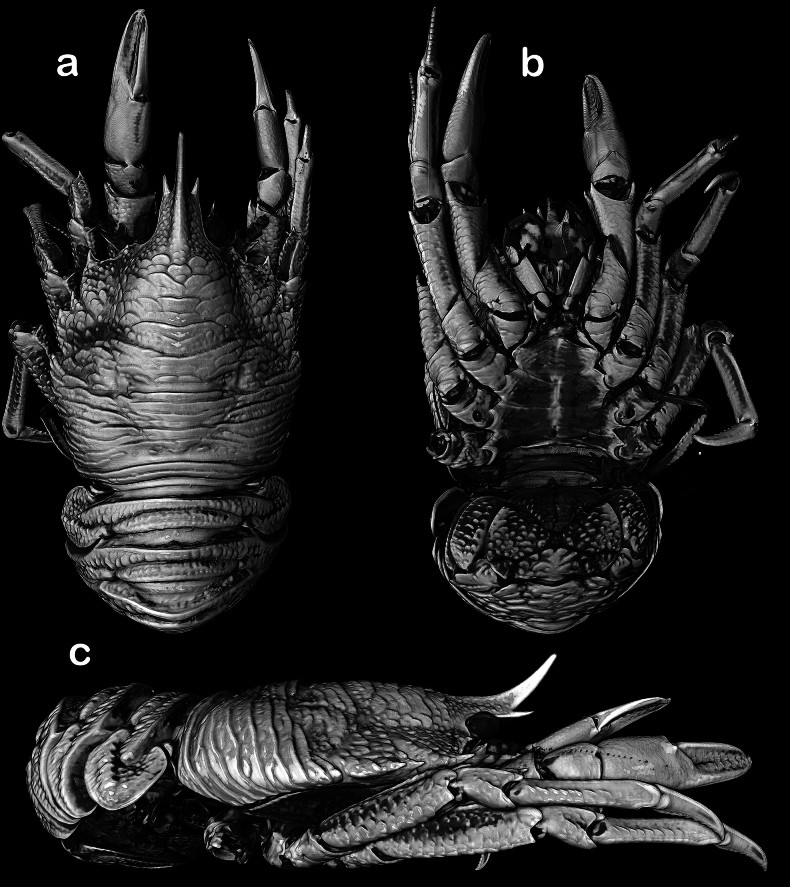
Munidopsisexpansa* Benedict, 1902, 3D rendering of micro-computed tomography x-ray images, F 17.7 mm PCL (MCZ CRU 11732), Cuba: A. Dorsal; B. Ventral; C. Lateral views.
